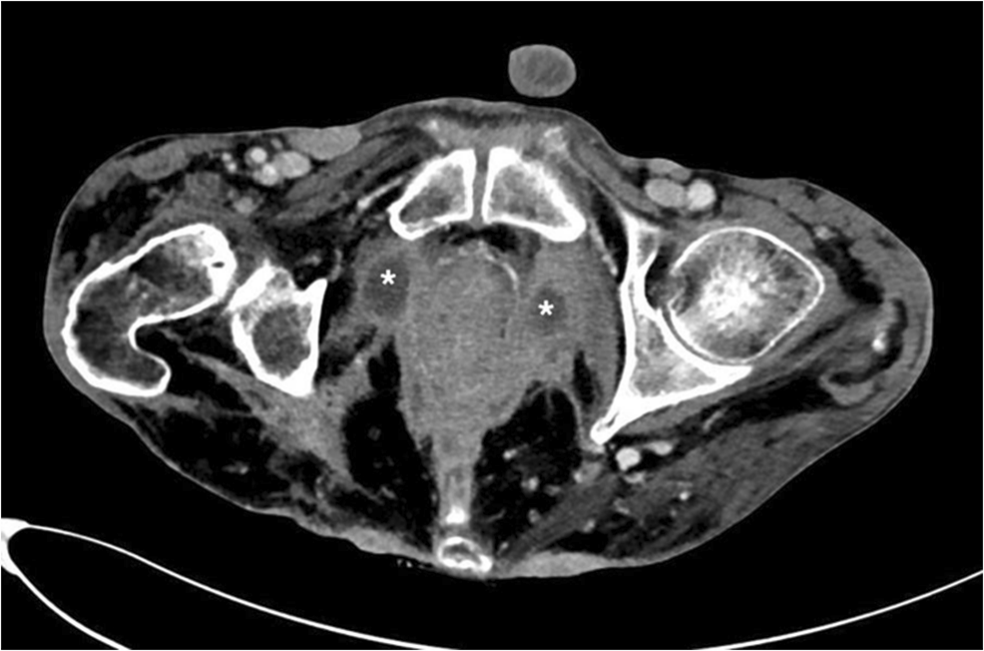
CT scan of the perineal and perianal region showing multiple abscesses (marked by an asterisk)
